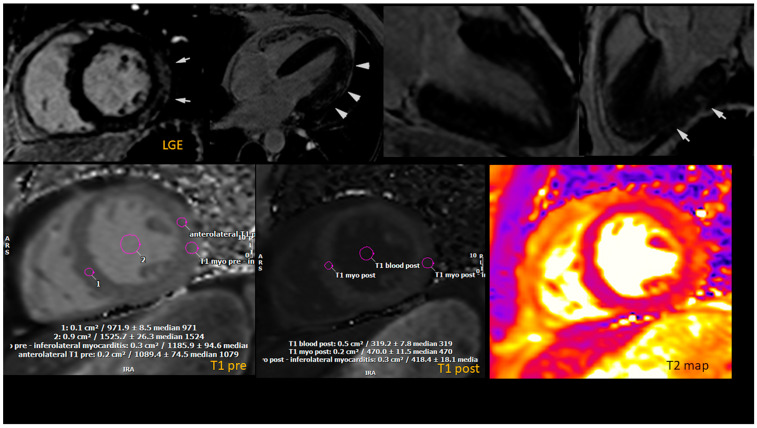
Cardiac magnetic resonance imaging findings. Top images demonstrate the presence of late gadolinium enhancement in lateral segments (white arrows). Bottom images demonstrate corresponding acute inflammation in basal lateral segments.
